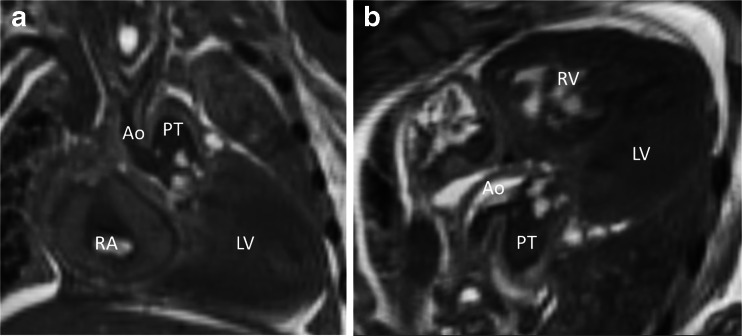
Neonatal cardiac postmortem MRI in a 10-day-old neonate who died unexpectedly at home. Coronal (a) and oblique axial (b) T2-weighted images show truncus arteriosus. The head and neck arterial vessels (*Ao*) arise anteriorly from the common trunk (*Ao/PT*) and the descending aorta posteriorly from the pulmonary arterial trunk (not shown). *LV* left ventricle*, RA* right atrium, *RV* right ventricle
